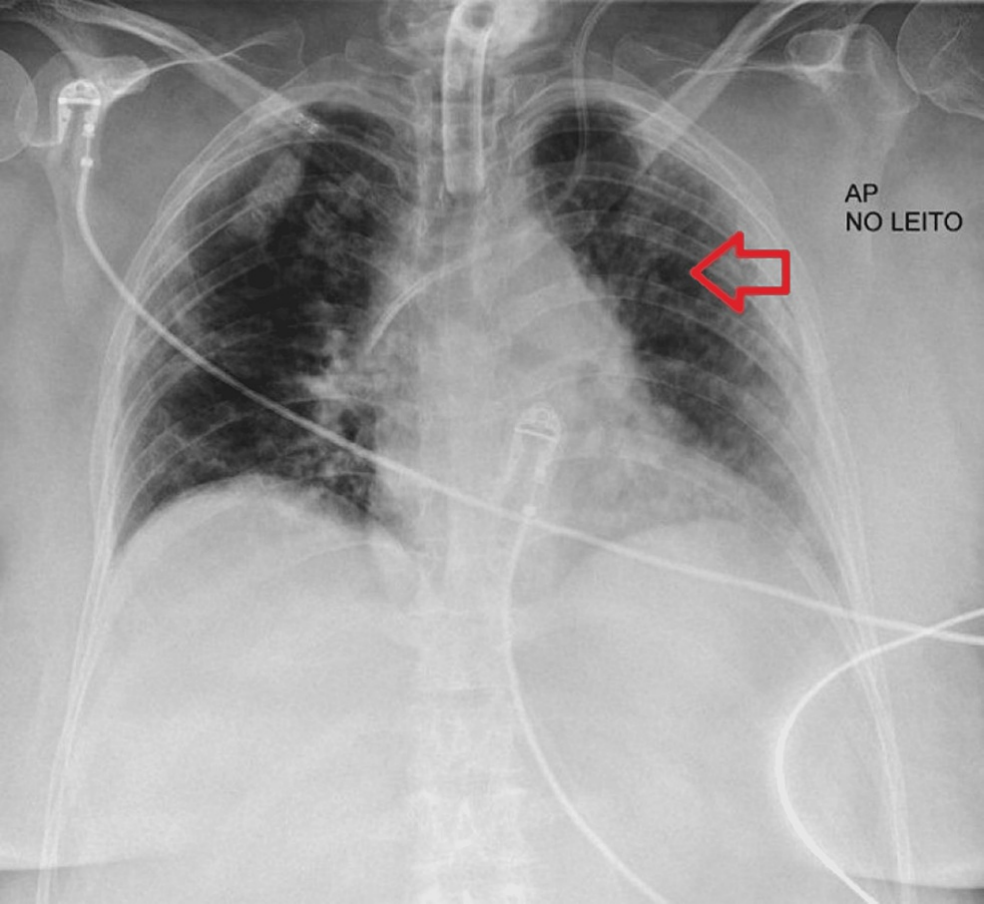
Chest radiograph obtained on the fifth day of methylprednisolone administration The image shows improvement of lung opacities (red arrow)
